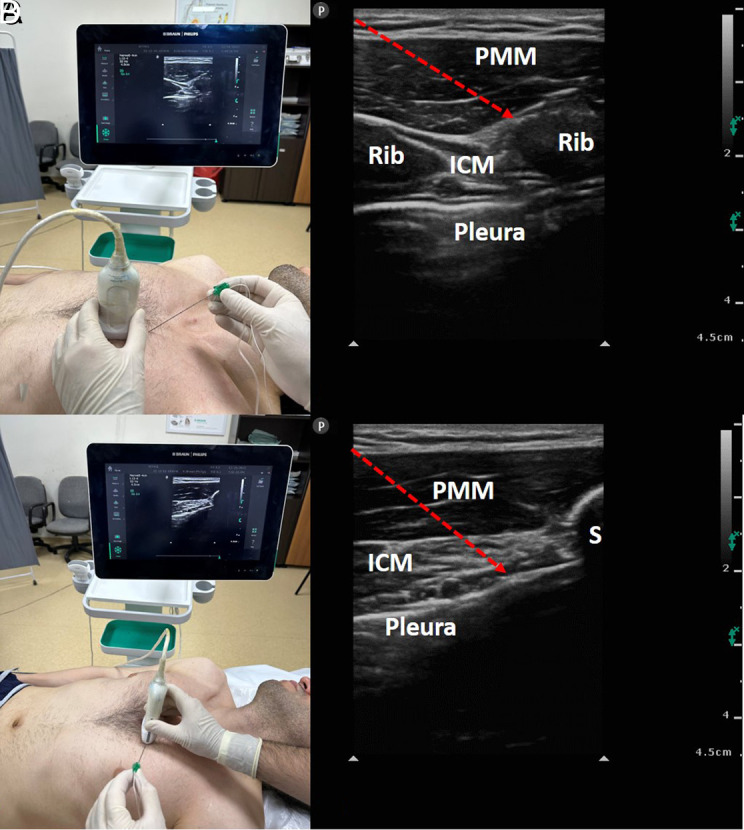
(A) Patient and ultrasound probe position for superficial parasternal intercostal plane block. (B) Sonographic anatomy of the superficial parasternal intercostal plane block. (C) Patient and ultrasound probe position for deep parasternal intercostal plane block. (D) Sonographic anatomy of the deep parasternal intercostal plane block. PMM, pectoralis major muscle; red arrow, needle direction.
